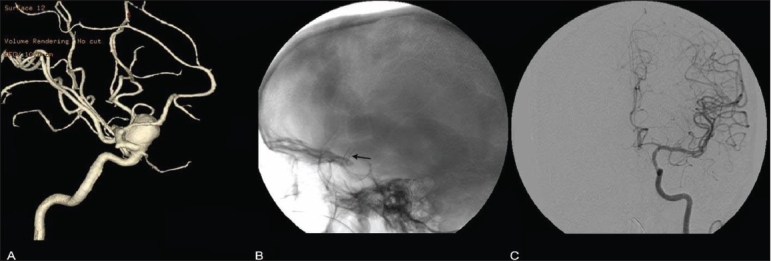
Stent-graft (cardiac covered stent) for a large ICA aneurysm. 3D rotation angiogram (A) shows an ICA aneurysm (aneurysm).A plain skull lateral radiograph shows the stent (arrow) across the aneurysm. Angiogram (C) shows post- stent non-filling of the aneurysm
